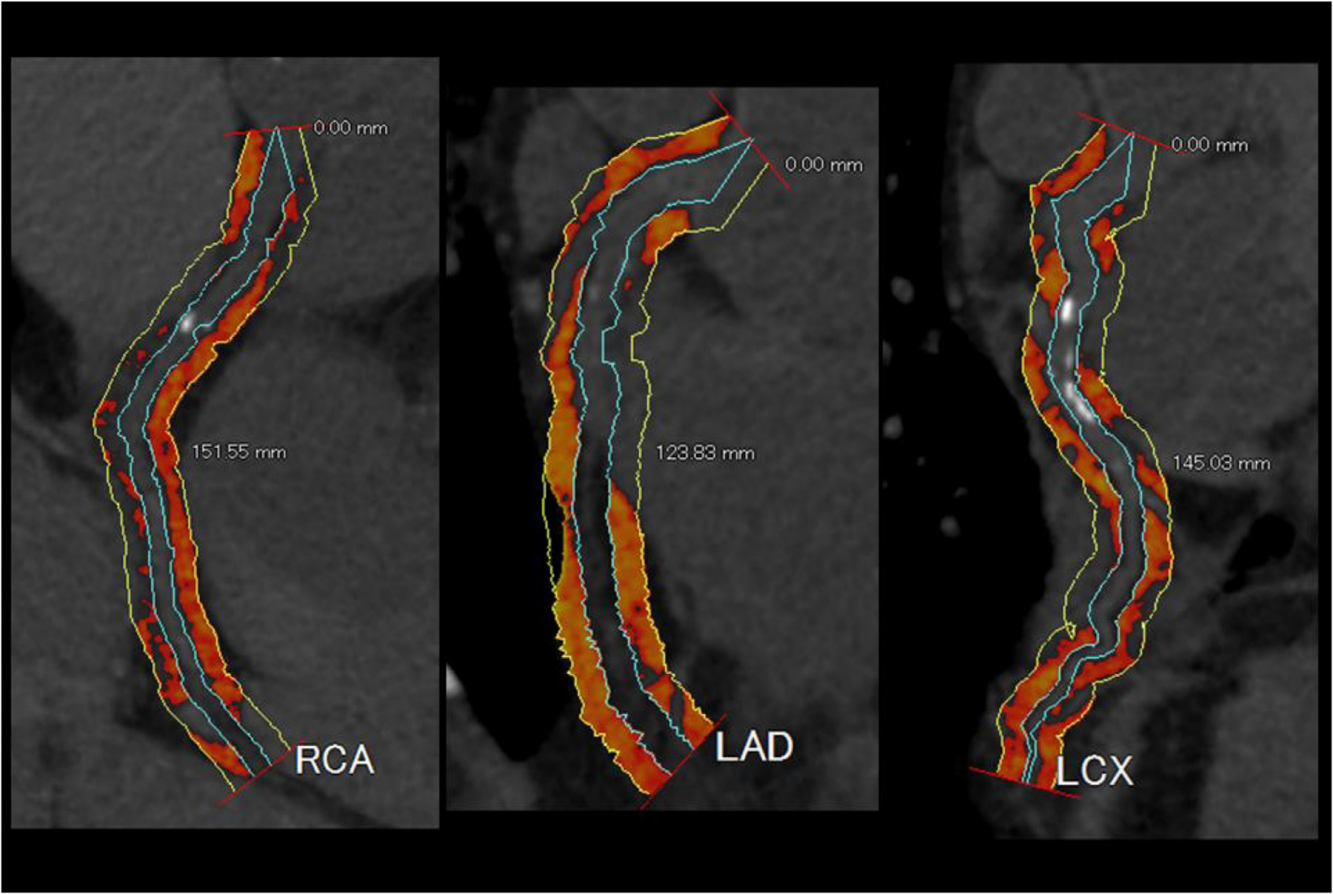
Perivascular fat attenuation index (FAI) analysis of coronary arteries. The light blue lines delineate the inner wall of the coronary arteries. The color maps show distribution of the CT numbers, where red indicates higher number and yellow indicates lower number. Abbreviations: RCA, right coronary artery; LAD, left anterior descending artery; LCX, left circumflex artery.
